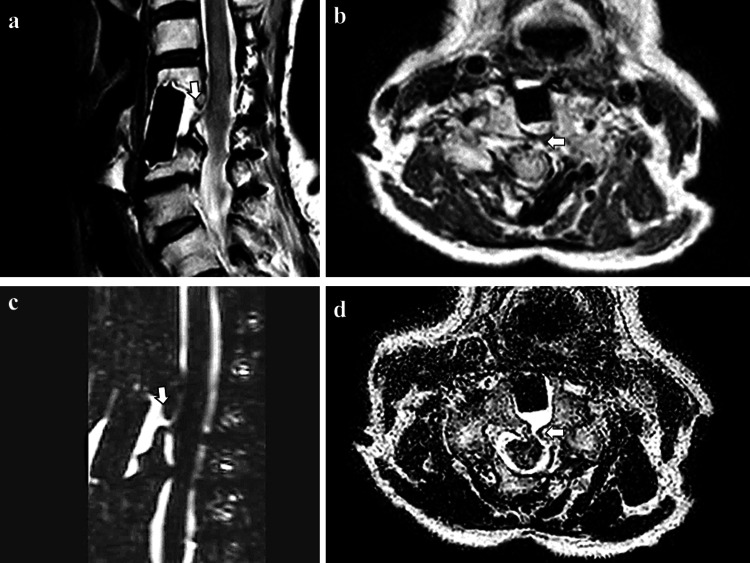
Cervical MRI (a) Sagittal T2-weighted cervical MRI and (b) axial T2-weighted cervical MRI showing a dural defect at the cervical level, which was a postoperative lesion of cervical discectomy; (c) Heavily T2-weighted cervical MRI showing the inflow of water into the dural fistula to the surgical discectomy and fusion lesion; (d) Axial constructive interference in steady state spinal MRI showing the dural defect clearer than T2-weighted MRI (b).
